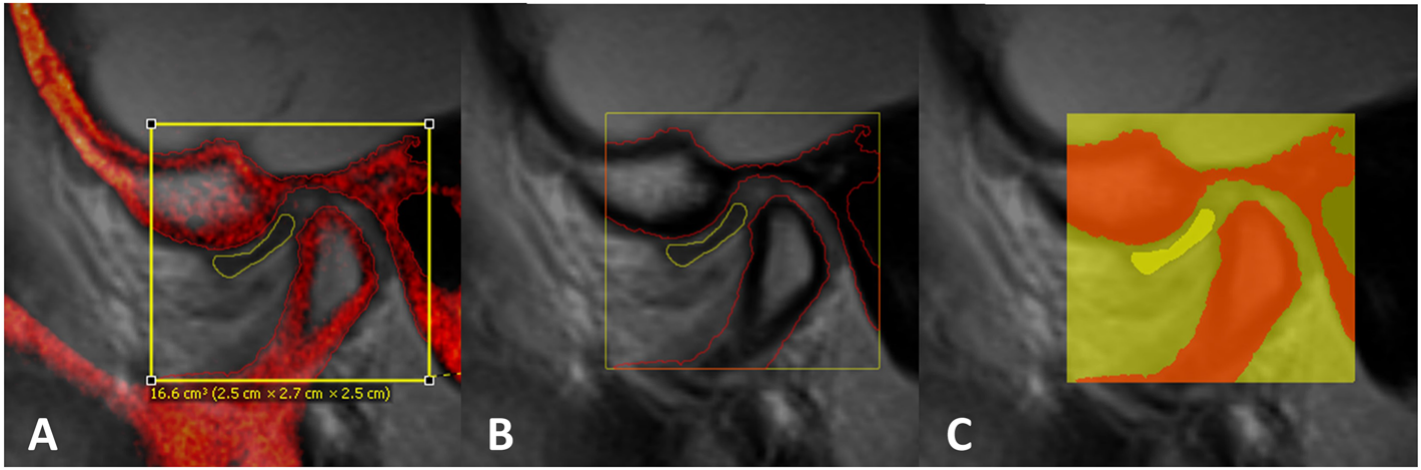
Process of segmentation. a Oblique sagittal PD-weighted MRI(*gray*)-CBCT(*red*) registered image showing 3D cropping box (2.5 × 2.7 × 2.5 cm^3^) that was manually drawn to include TMJ articular disc, condylar head, and temporal components. b Oblique sagittal PD-weighted MRI only showing the outlined osseous structures (*red*) and articular disc (*yellow*) from the co-registered CBCT image. c Same image as B. with highlighted cropped structures to be exported as STL files
